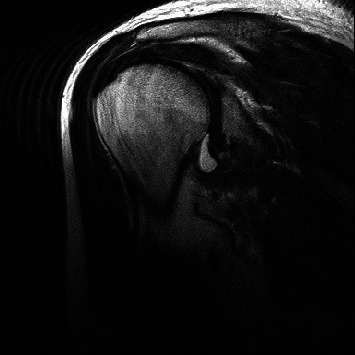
Magnetic resonance imaging coronal view demonstrating disruption and fluid accumulation at insertion of right latissimus dorsi tendon.
